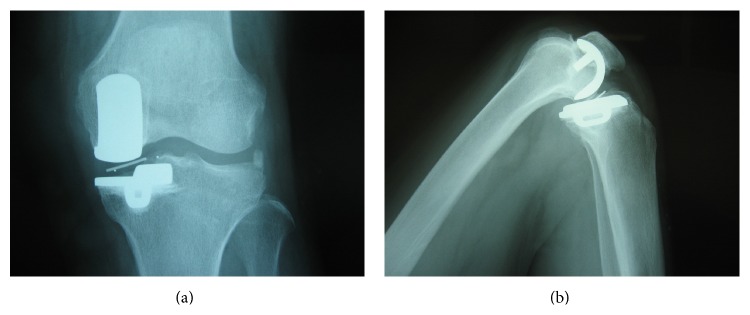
Anteropoasterior (a) weigh-bearing radiograph and lateral (b) radiograph in knee flexion showing the abnormal position of marker in meniscal bearing (case 1).
